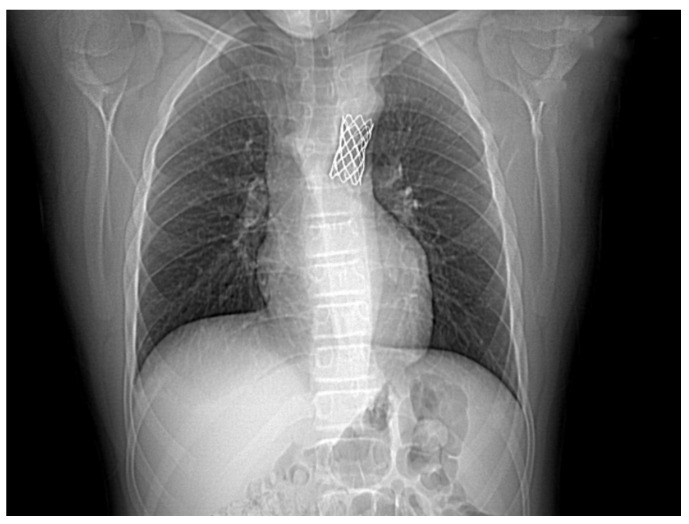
Control native chest CT visualizing the correct position of the stent.
